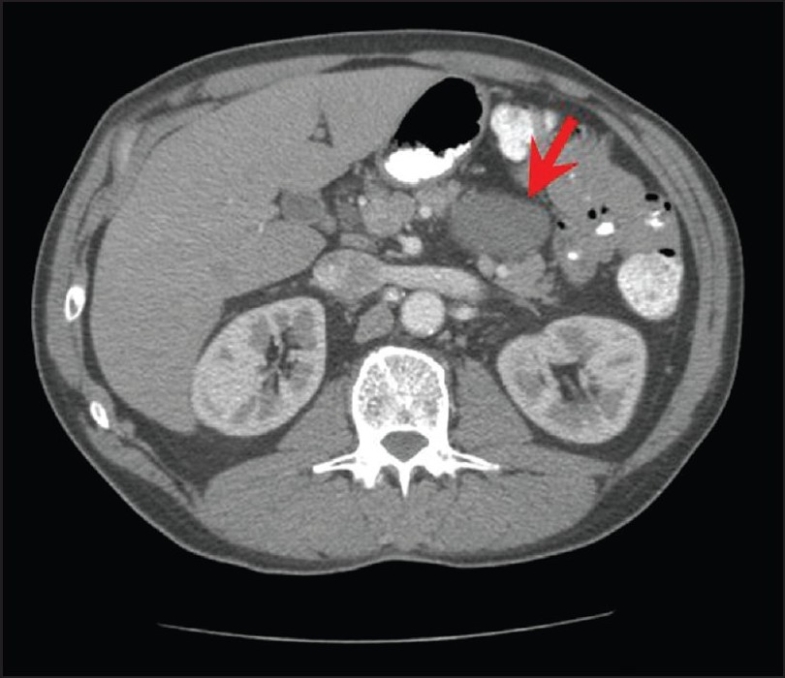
CT scan of the abdomen shows a 5.3 × 4.4 cm cystic lesion (arrow) in the body/tail of the pancreas without any changes in acute or chronic pancreatitis
